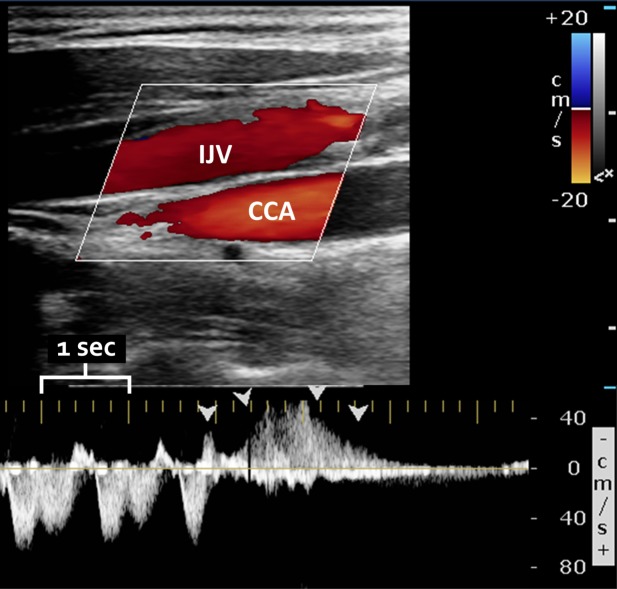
Sample color (top) and spectral (bottom) Doppler ultrasound of the internal jugular vein (IJV), demonstrating reflux (with the same direction of flow as the underlying common carotid artery [CCA]). The large yellow tick marks indicate 1-second intervals. Arrowheads demonstrate reflux lasting >0.88 seconds. Abnormal reflux was only determined using the spectral Doppler waveform, which allows precise determination of flow direction and duration.
